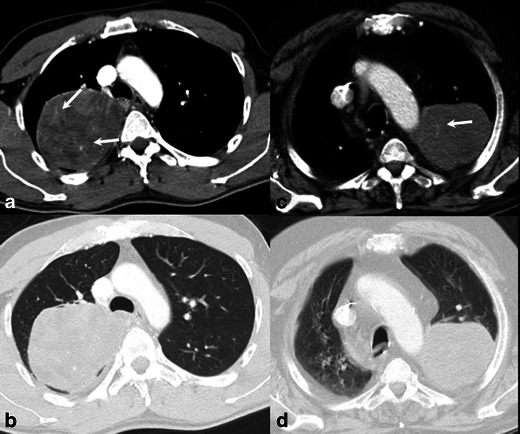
CT images of primary pulmonary sarcomas. A 39-year-old man (a and b) with synovial sarcoma in the right upper lobe, mass of ovoid shape, smooth margins, heterogeneous enhancement and prominent intratumoural vessels (*arrows* in a). A 86-year-old man (c and d) with angiosarcoma, also showing a large mass with smooth margins and identifiable intratumoural vessels (*arrow* in c)
